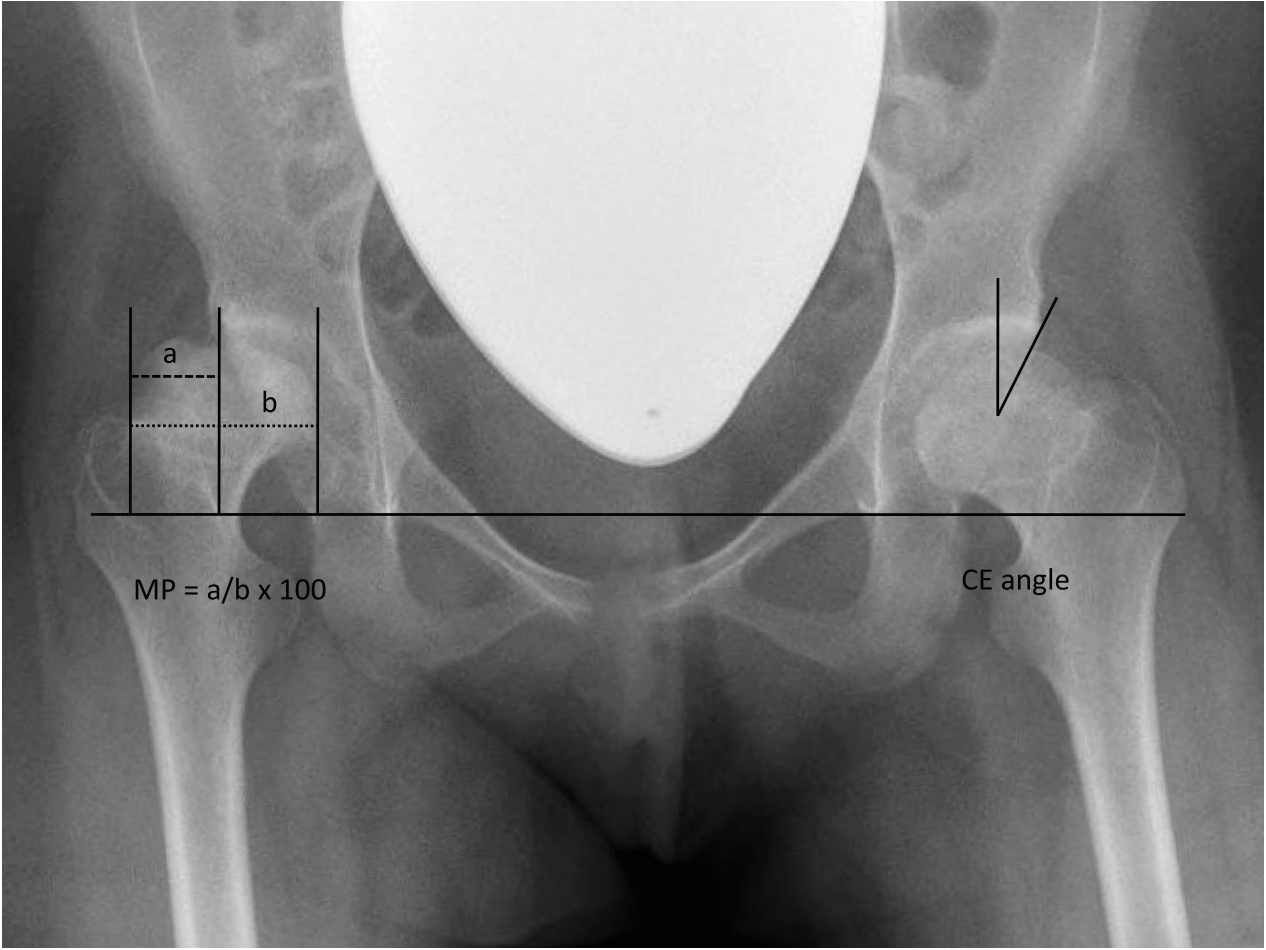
Radiograph of a 15-year-old severely affected female patient demonstrating measurement of the Reimers percentage (MP, right hip) and Wiberg's center-edge angle (CE, left hip).
